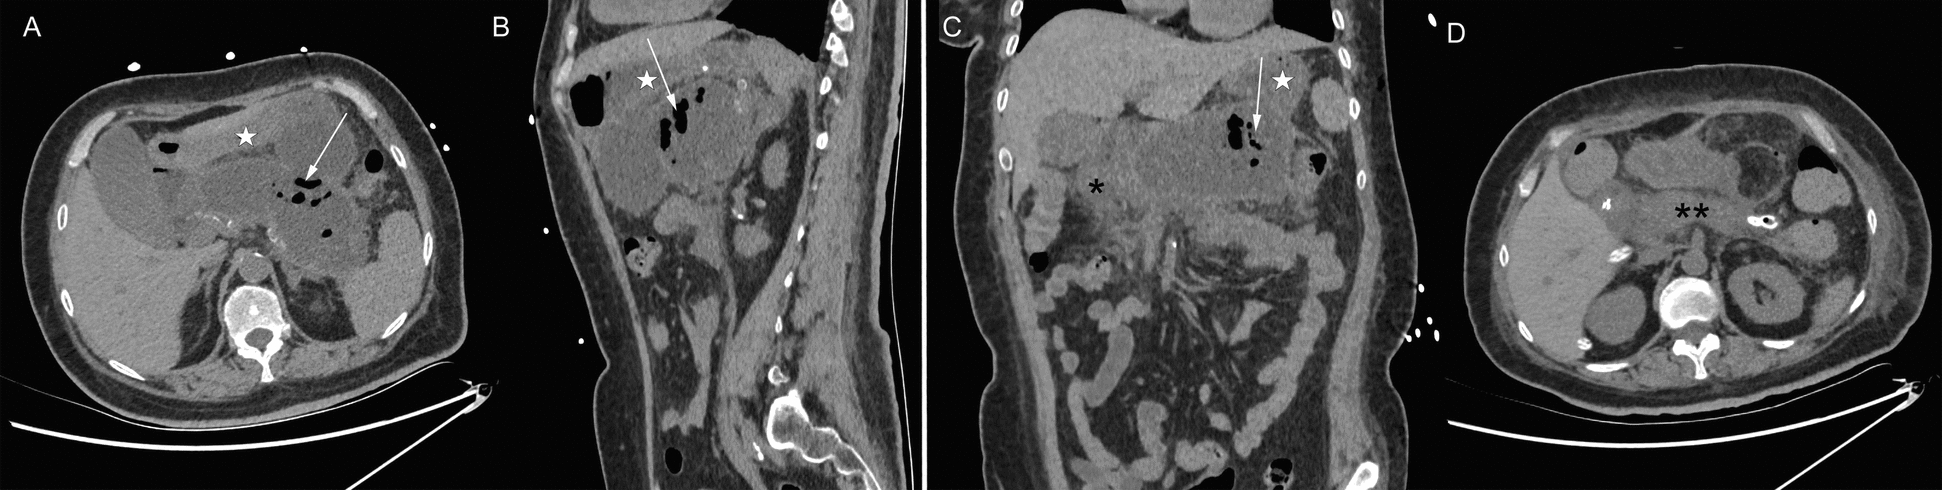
Abdominal computed tomography scan during the perioperative period of the pancreatic necrosectomy. Axial (A), sagittal (B), and coronal (C) computed tomography showing gas formation (arrows) within the walled-off peripancreatic necrotic collection on postoperative day 4 after parathyroidectomy (hospital day 10). D resolution of peripancreatic collections on postoperative day 12 after necrosectomy; stars, stomach; asterisk, duodenum; double asterisk, pancreas
